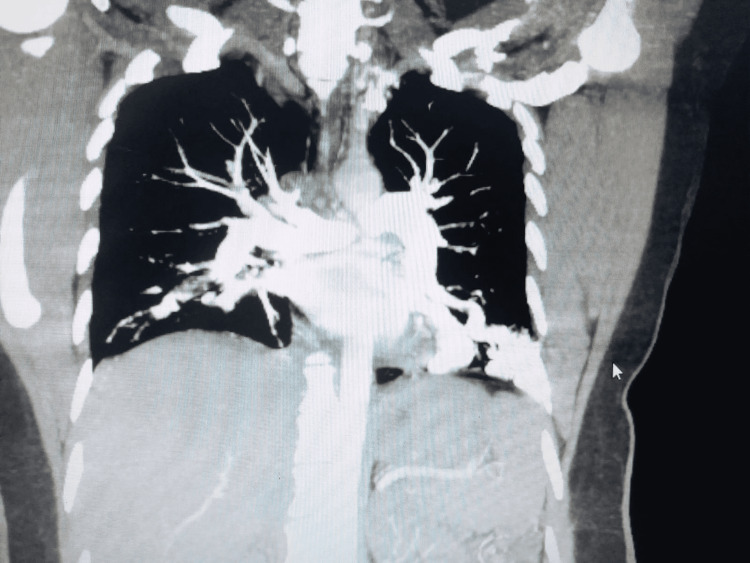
Coronal contrast CT image showing arteriovenous (AV) malformations Coronal contrast reformatted CT image, set on the mediastinal window. Maximum intensity projection (MIP) software was applied to the images to make the vascular connections more conspicuous.
